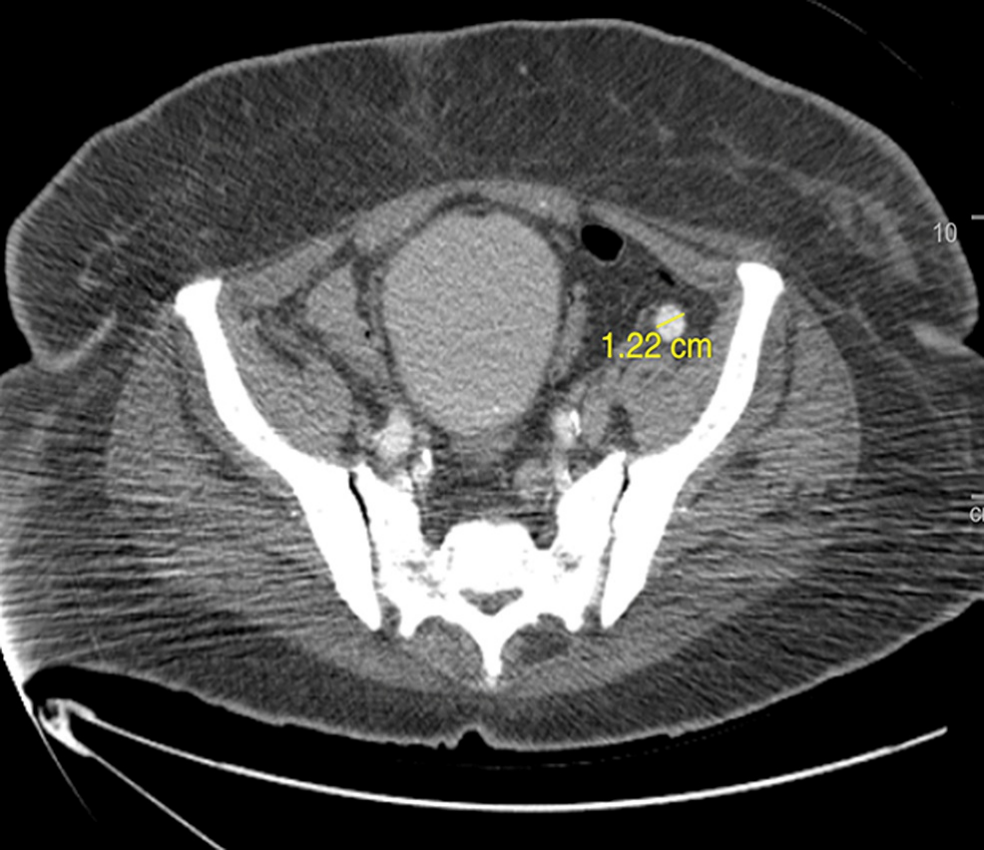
Axial CT scan of the pelvis. Axial CT scan showing enlarged right external iliac lymph node measuring 1.22 cm in diameter.
